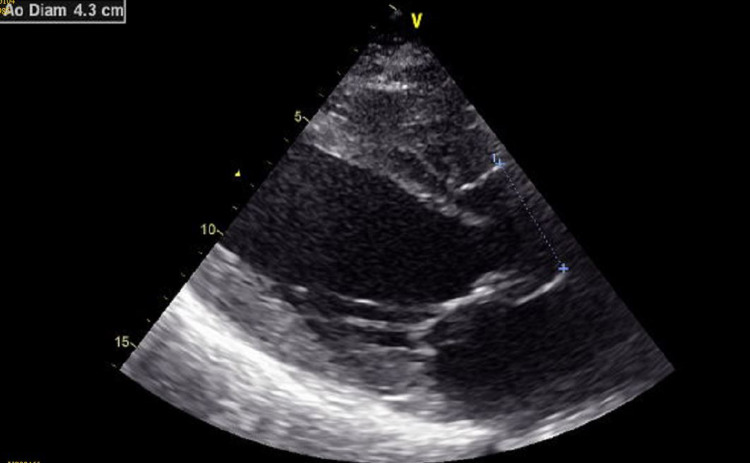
Parasternal long axis view showing dilatation of the ascending aorta.
